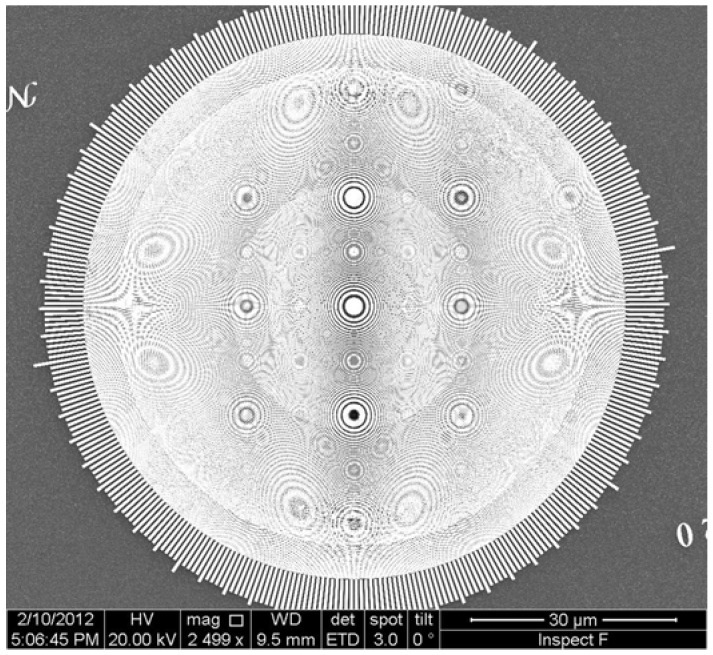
Overall pattern of one of the most advanced hard-X-ray FZPs of today, with an outermost zone width of 20 nm [1].
